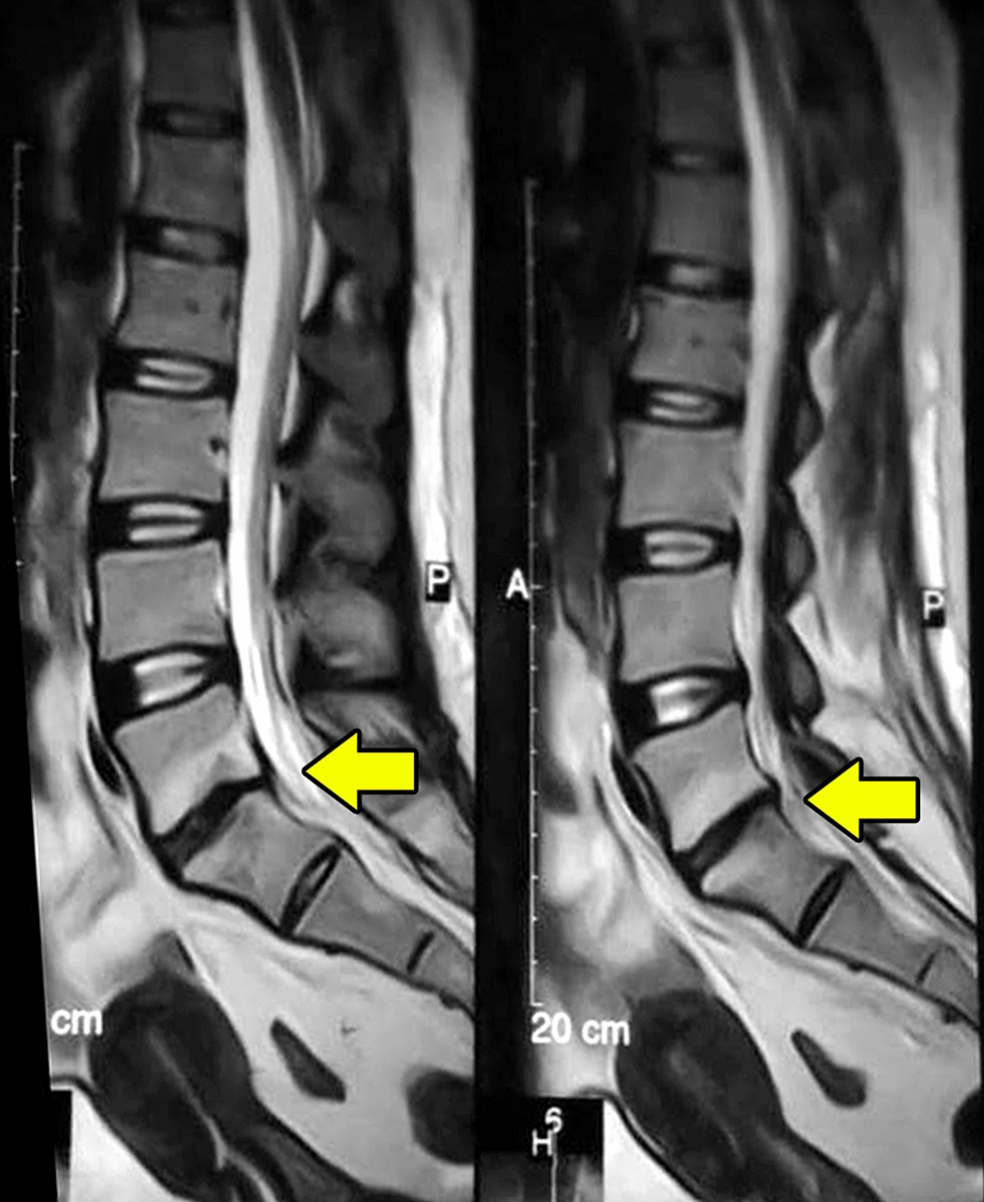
Post-treatment MRI images. Two sagittal sections of the T1-weighted MRI after six weeks of antibiotic treatment. The imaging showed complete resolution of the discitis with the presence of endplate irregularities at the L5-S1 level.
